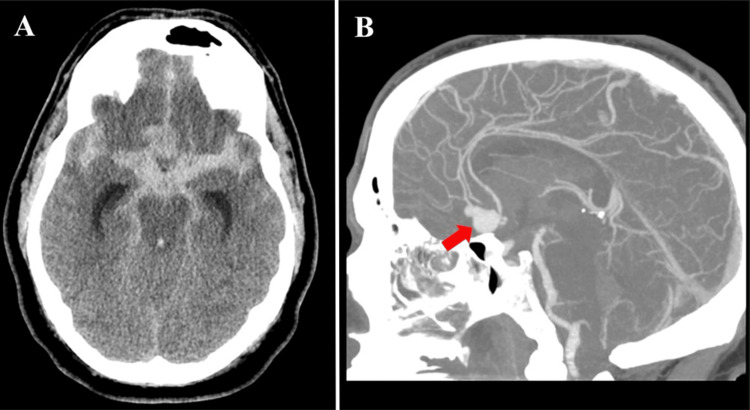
Non-contrast head CT and CT angiogram in the preoperative period showing a large anterior communicating artery aneurysm (A) Non-contrast head CT with extensive subarachnoid hemorrhage. (B) CT angiogram with a saccular aneurysm in ACom as depicted by the red arrow.
